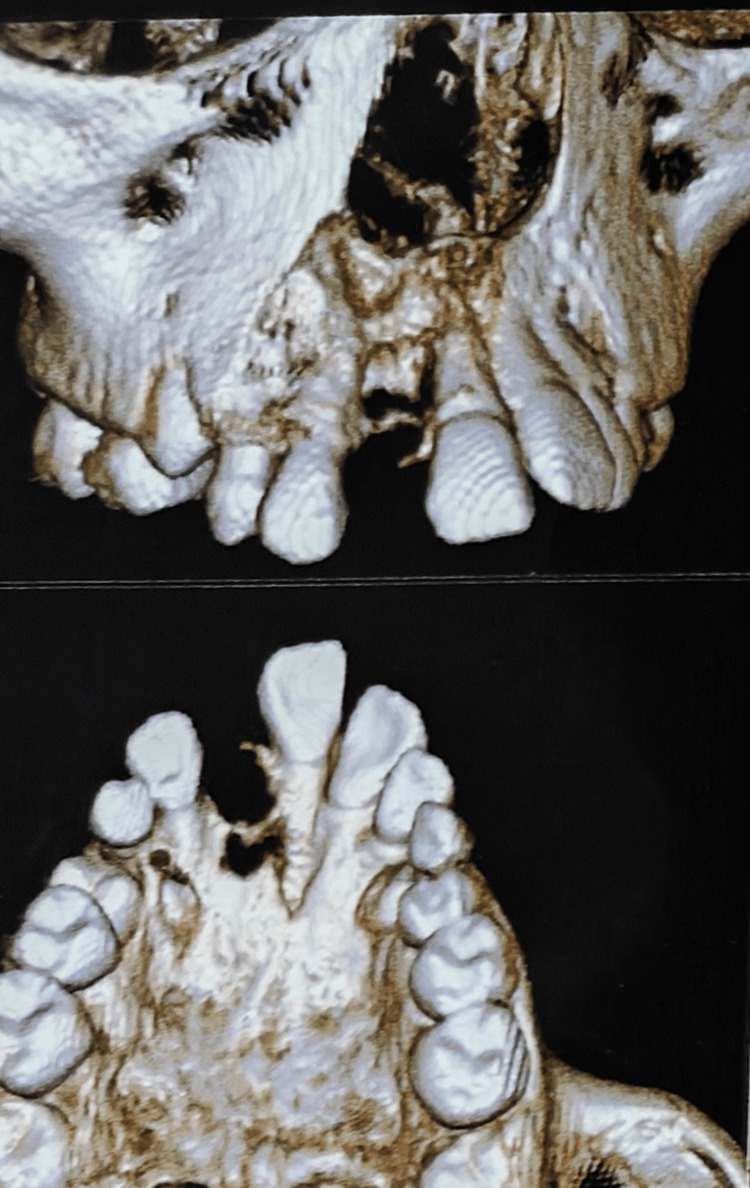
CBCT images showing bone destruction in the anterior maxilla. CBCT: Cone-beam computed tomography.
